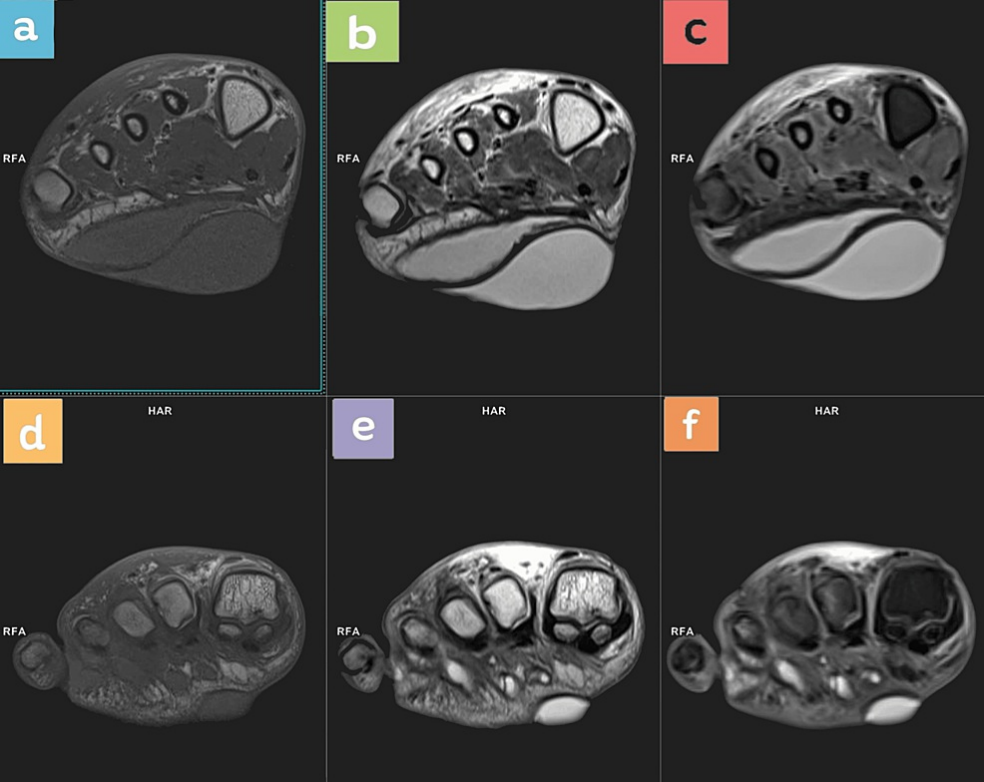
Coronal MRI sequences at the level of phalanges Top row (at the midfoot level): left to right: a) T1, b) T2, c) PD FS. Bottom row (at the level of the metatarsophalangeal joint): left to right: d) T1, e) T2, f) proton density fat saturated (PDFS).
